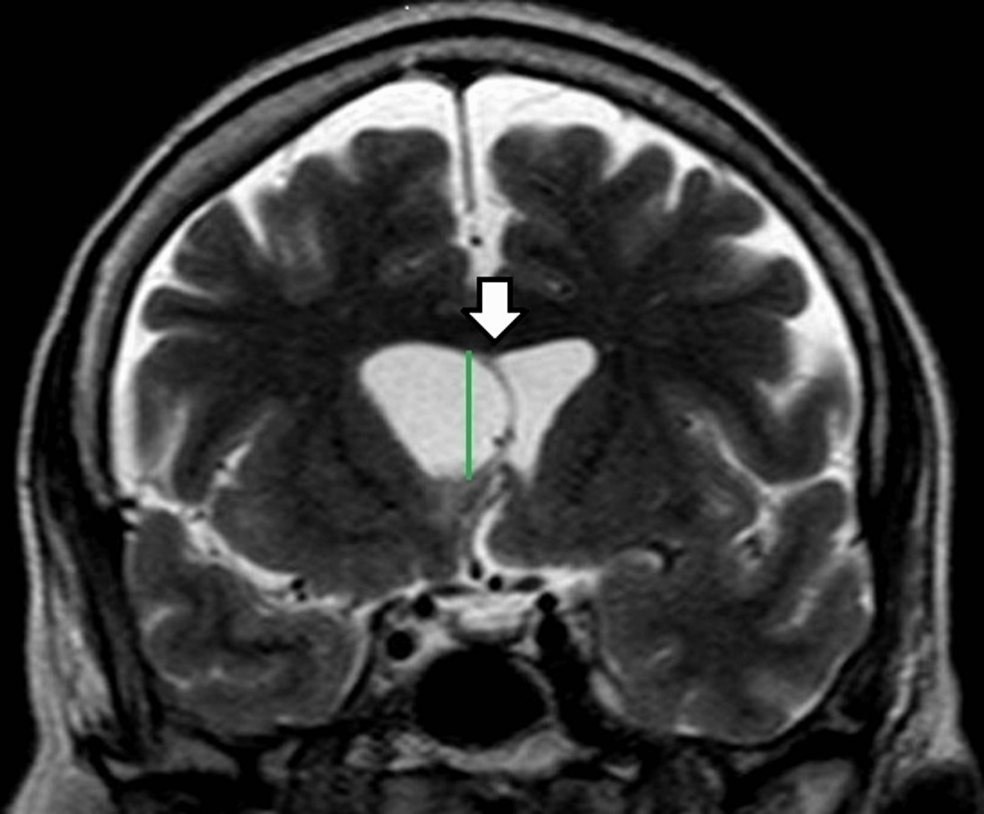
Brain T2-weighted MRI showing dilatation of the ventricular system and deviation of the septum pellucidum to the left (arrow showing the septum pellucidum and green line showing midline)
